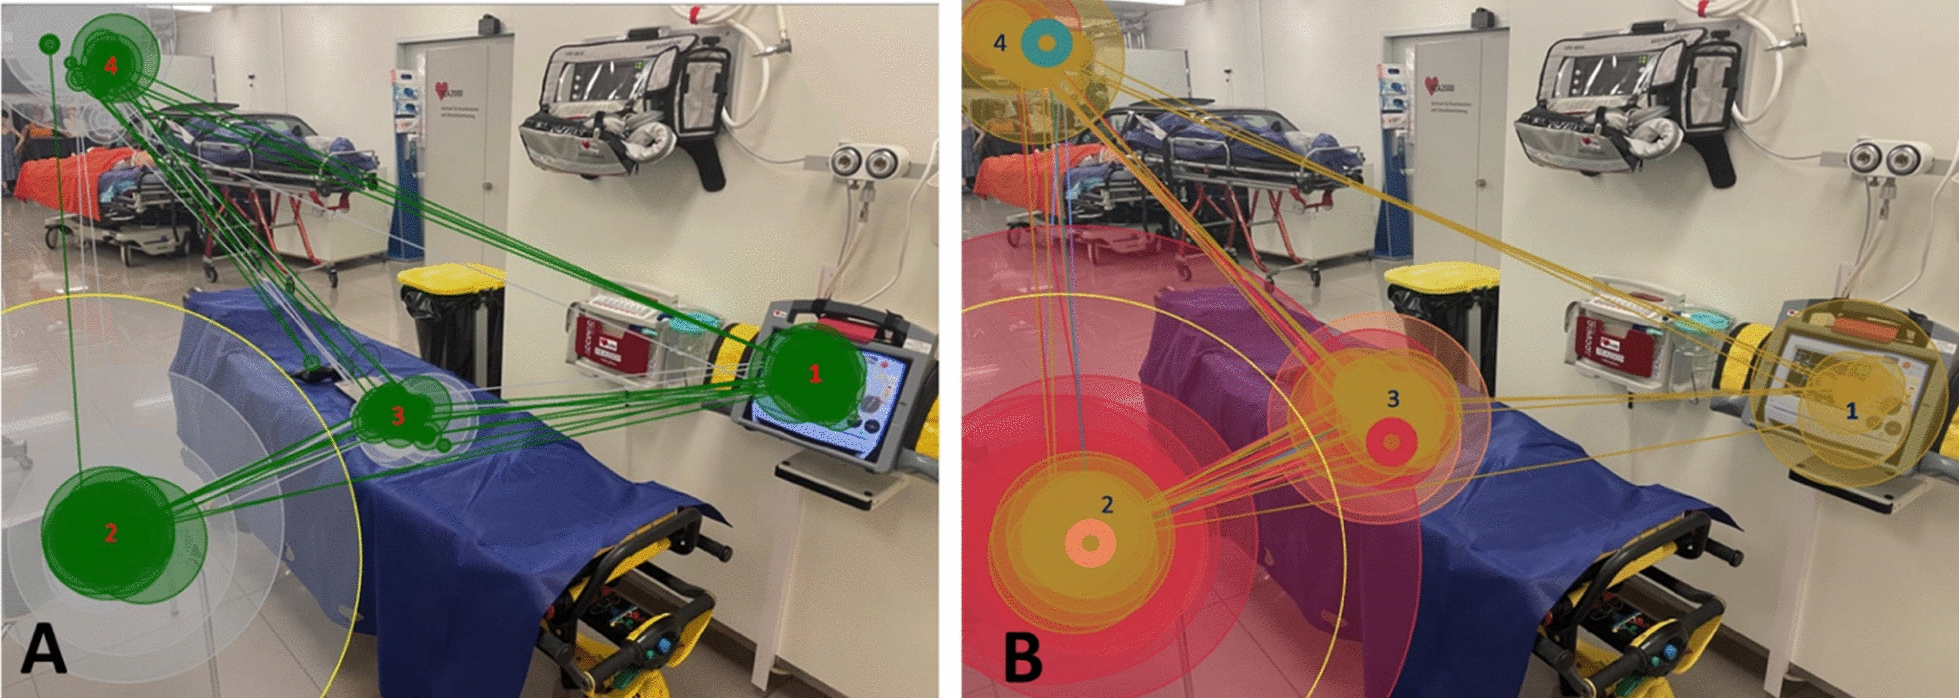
Heatmap of overall gaze behavior. Areas of interest: 1 = patient monitor, 2 = ultrasound screen, 3 = patient, 4 = blank (irrelevant fixations, e.g. on the floor). A Heatmap of ‘red’ group (i.e. participants who recognised desaturation); B Heatmap of ‘blue’ group (i.e. participants who did not recognise desaturation)
